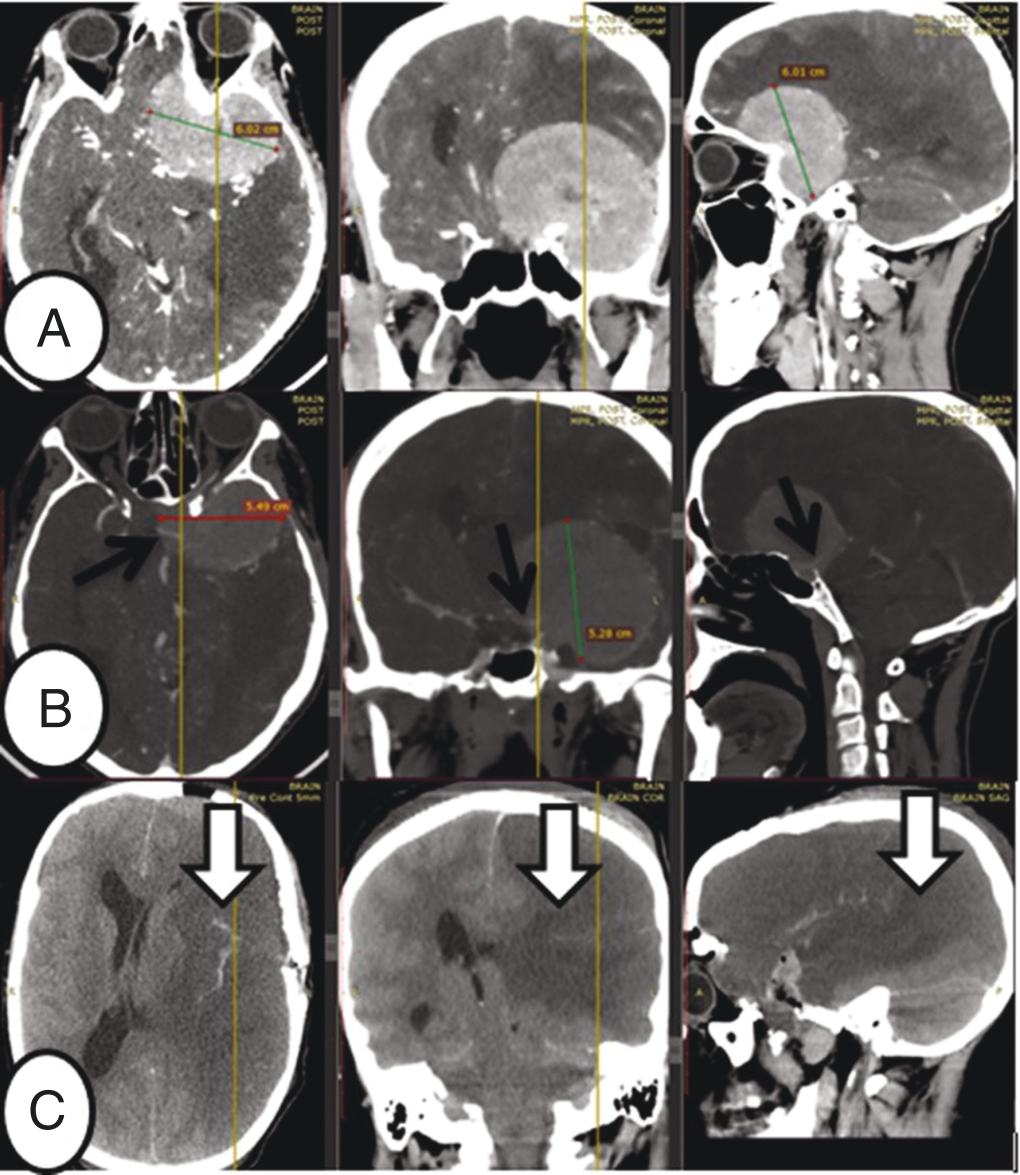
This figure depicts the image of a patient who was diagnosed to have left cerebral infarction secondary to iatrogenic middle cerebral artery (MCA) injury during surgery for a left-side clinoidal meningioma. The image in (A) was a preoperative CT scan with contrast of a patient and showed giant left clinoidal meningioma. The arrow in (B) depicted the CT angiography of a patient who had a 360-degree encasement of the MCA by the tumor. Follow-up CT was obtained for the same patient within 6 hours of the completion of the surgery. The down-pointing arrows as depicted in (C) showed left MCA infarction due to iatrogenic injury of MCA.
